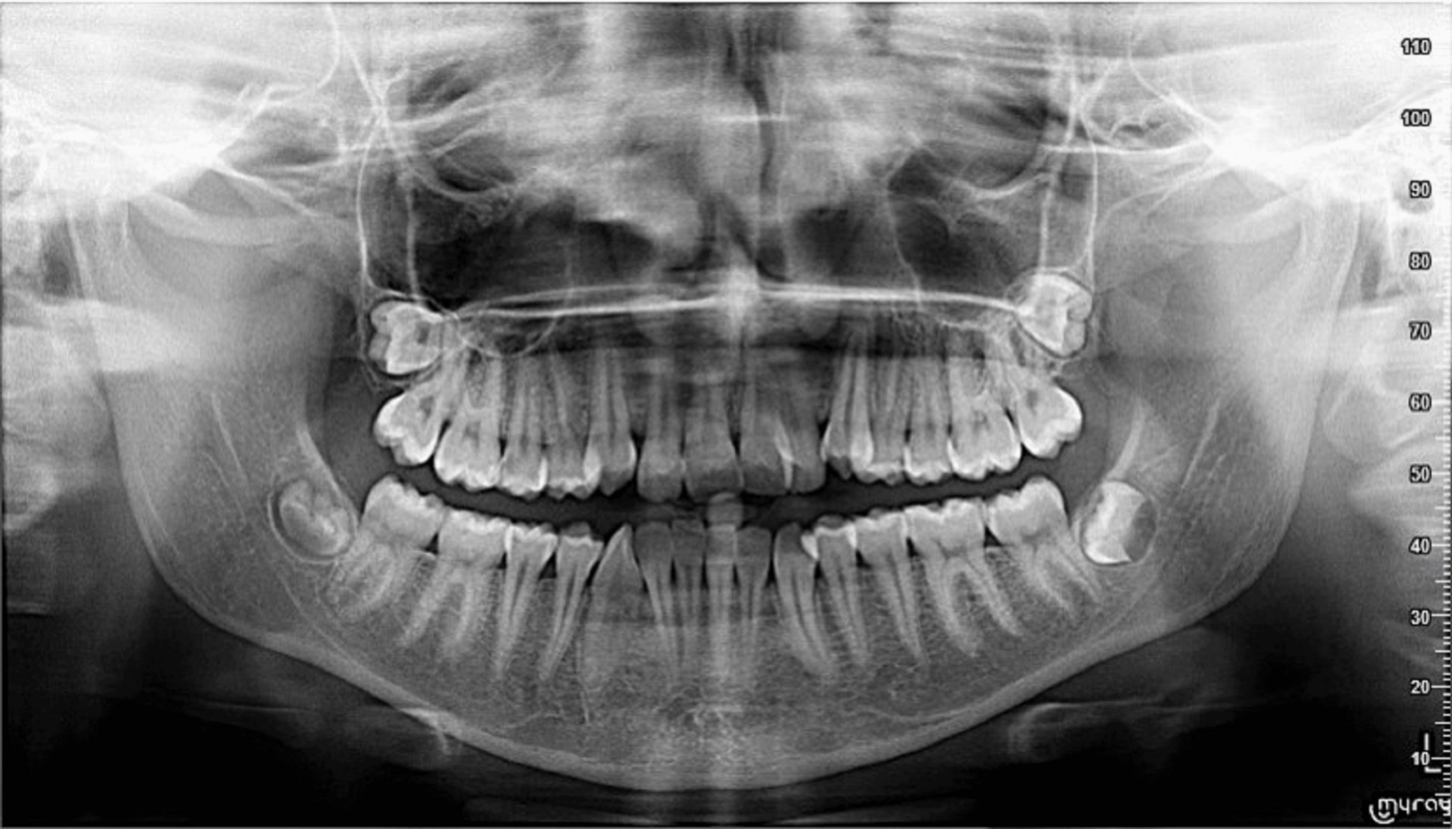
Panoramic radiograph taken before treatment. Panoramic radiograph showing the overall dentition and supporting structures.
